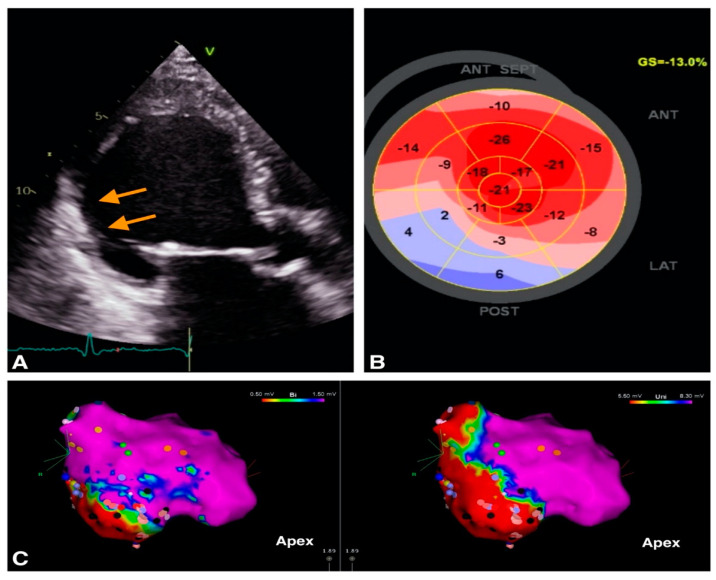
Example of image integration from a patient with VT and old myocardial infarction; (A). 2D apical 3 chambers view showing the thin, hyperechogenic inferolateral wall (orange arrows). (B). Bull’s eye representation of peak longitudinal strain showing positive values in inferior wall providing a description of the myocardial scar (C) Electroanatomic bipolar (left) and unipolar (right) voltage maps in septal view, with an inferior area of low voltage corresponding to the scar identified by echocardiography.
